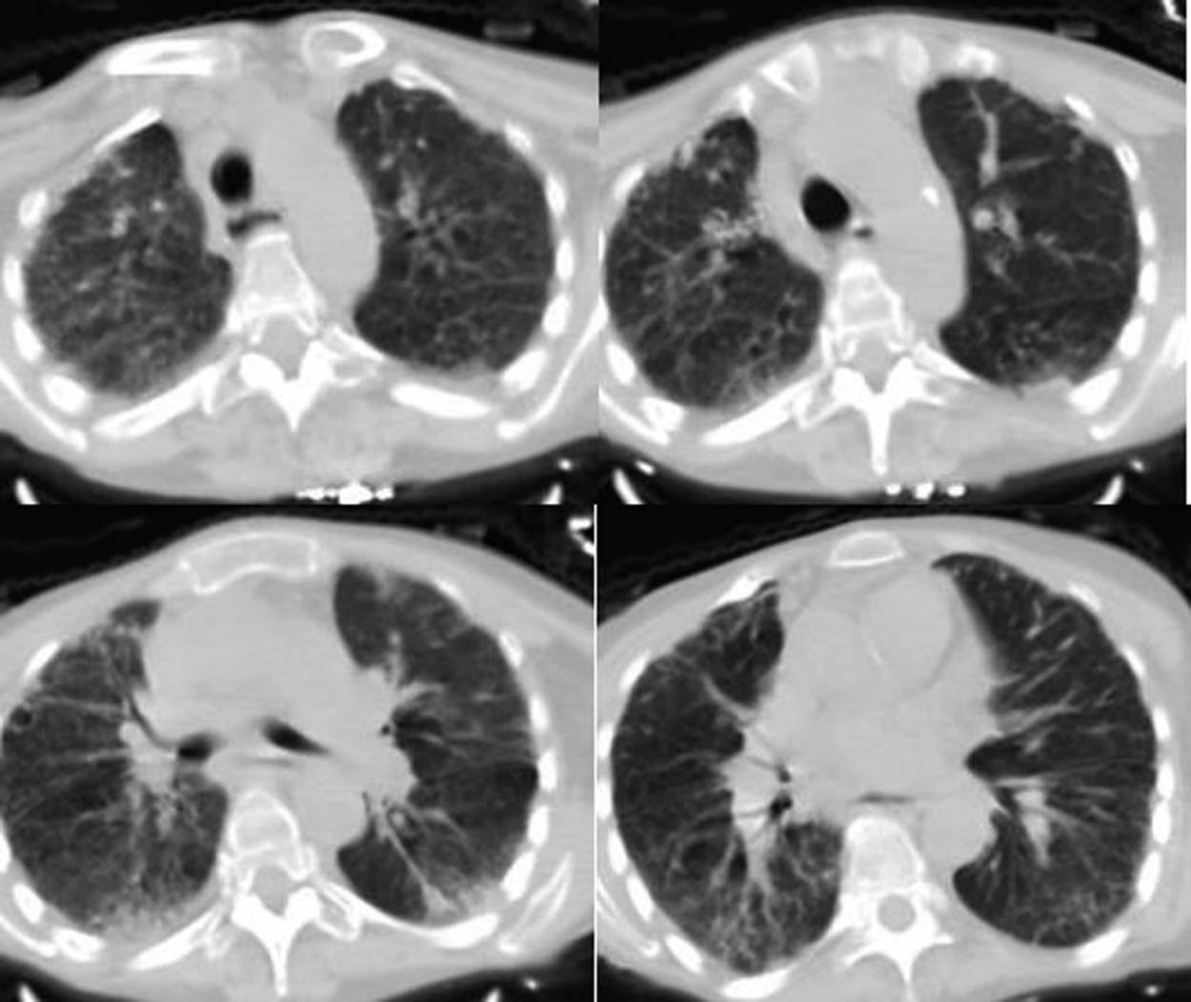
A chest CT scan, showing the interstitial pulmonary fibrosis. CT, computed tomography.
